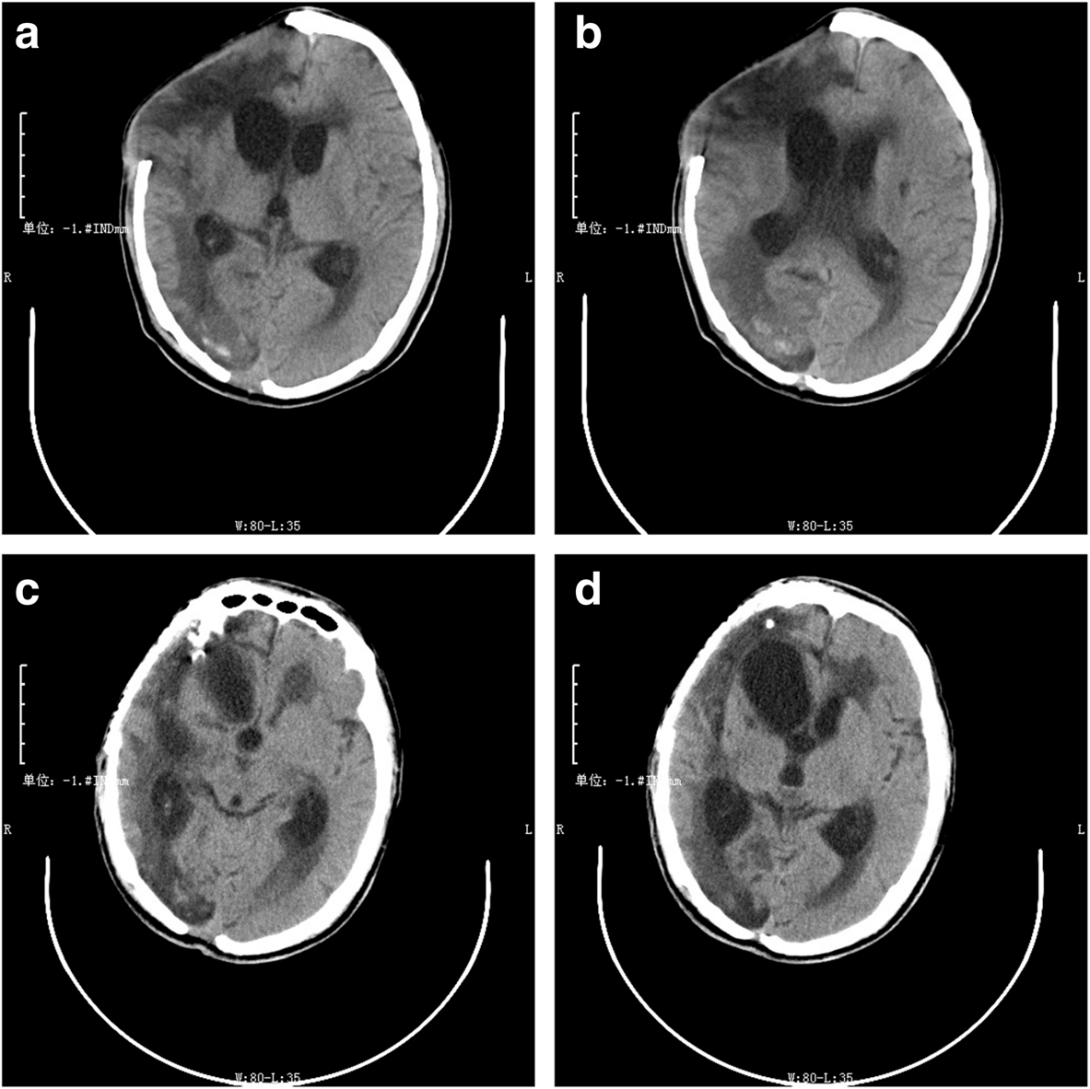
Two months after discharge, a head computed tomography (CT) scan a and b revealed hydrocephalus. c and d show the head CT scan of the patient 1 month after the repair of the skull defect
